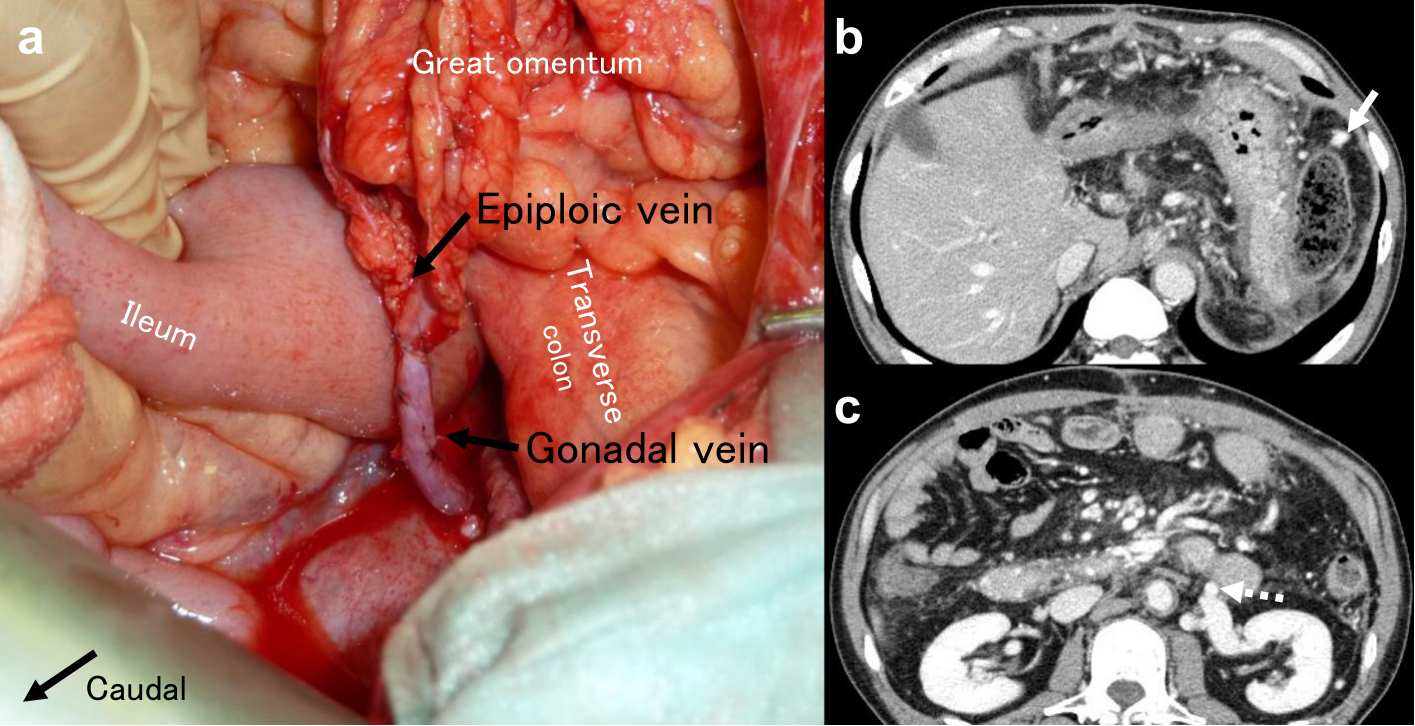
Epiploic gonadal bypass. a Intraoperative photograph showing end-to-end anastomosis of the epiploic and left gonadal veins. b, c Postoperative computed tomography showing patent and well-dilated epiploic vein (*solid arrow*) and left gonadal vein (*dotted arrow*)
